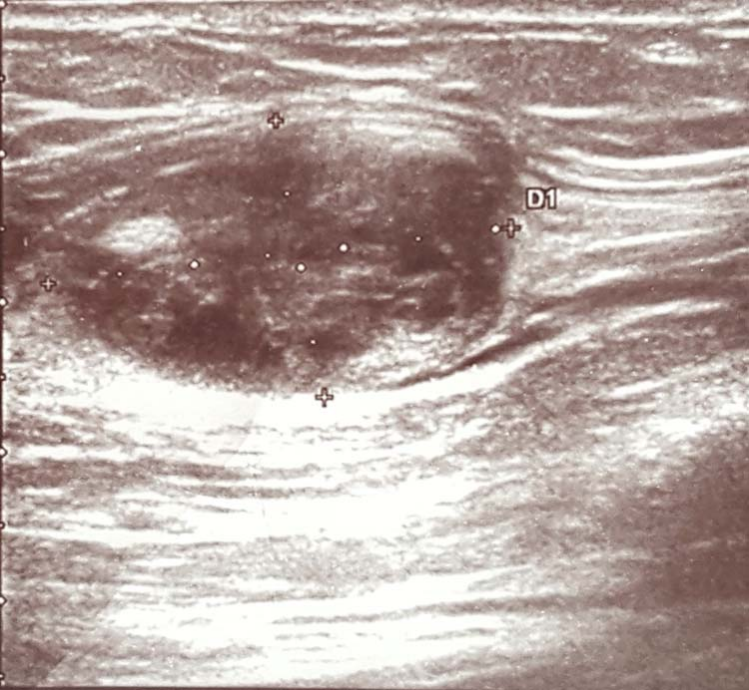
Ultrasound showing a heteroechogenic mass in the left arm.
